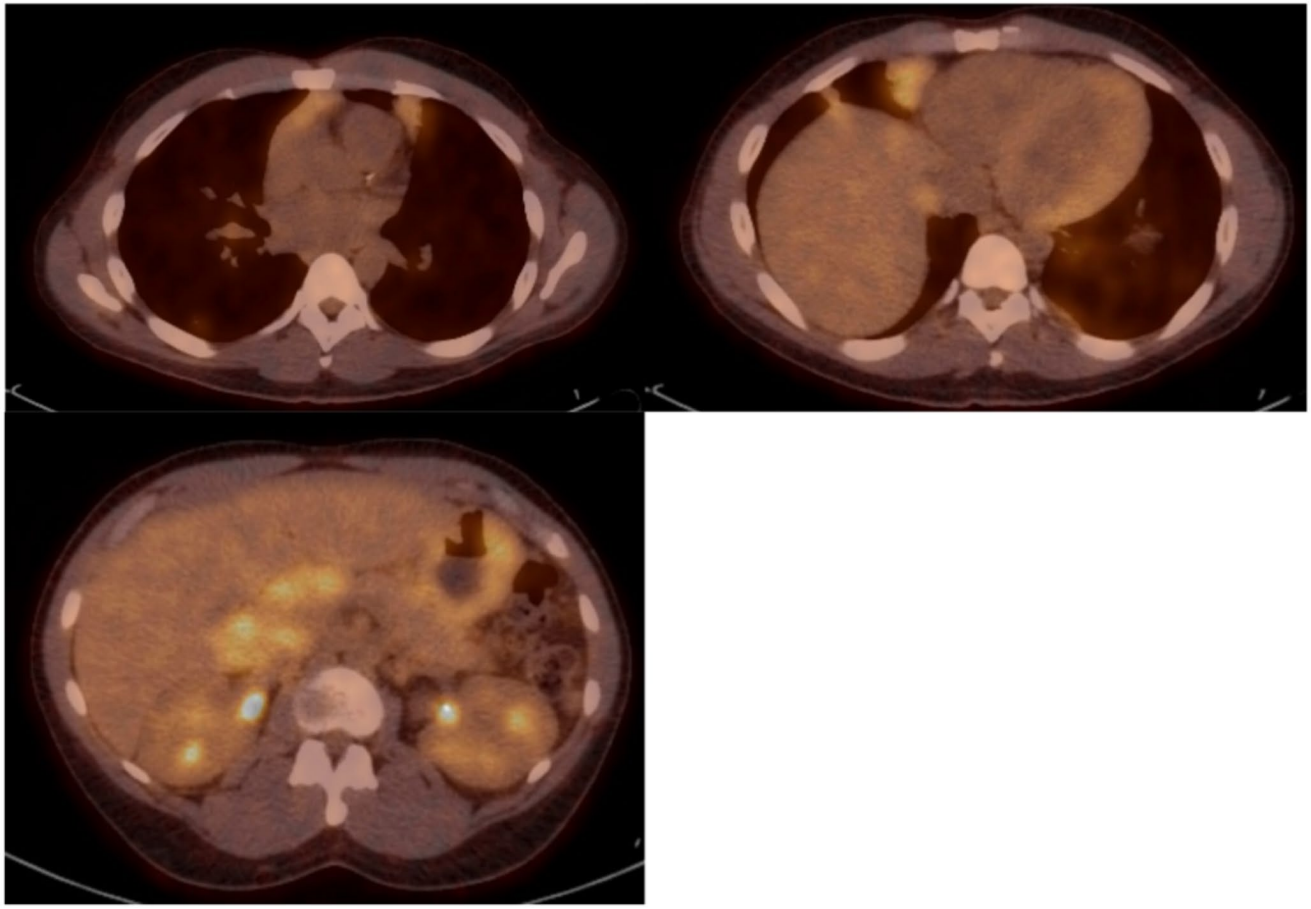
PET CT-study. A large anterior mediastinal mass with extension to the thoracic inlet, mediastinal lymph nodes, liver lesions, and periportal lymph nodes near the head of the pancreas were demonstrated
